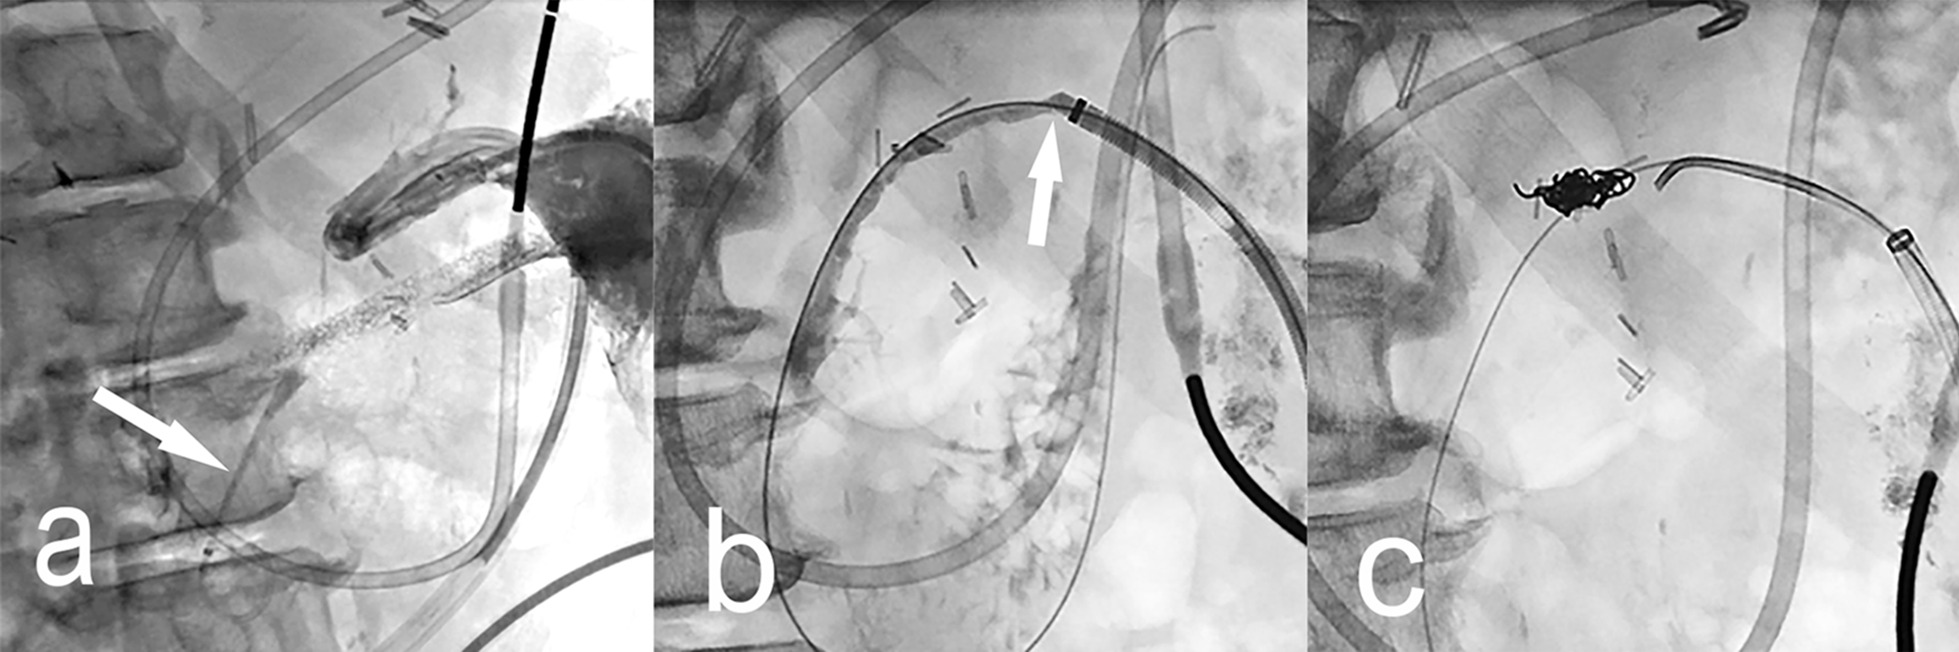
Disconnected pancreatic duct syndrome managed with transcavitary duct stent placement. Patient 8 presented with severe necrotizing pancreatitis. a Coronal reconstruction of abdominal CT scan shows a very large peri-pancreatic walled-off necrosis (WON). b Abdominal radiograph during contrast injection into the WON cavity 2 weeks of drainage shows improvement but persistence of amorphous filling defects caused by debris (arrowheads). c Radiograph during percutaneous necrosectomy shows a forceps introduced through a peel-away sheath grasping the large conglomerates of necrotic and fibrinous material during the process of evacuation. d After resolution of the peri-pancreatic WON a cavitygram shows communication with the pancreatic duct (arrowheads) through a focal disruption (curved arrow) at the neck of the pancreas. e Plastic stent in transit through the percutaneous tract is faintly visible as it is advanced in a folded configuration (arrowheads) over parallel guidewires, one directed towards the pancreatic tail and the other through the ampulla. f CT scan shows the plastic stent appropriately positioned in the pancreatic duct bridging the head and tail segments of pancreatic duct. After one month the stent was removed endoscopically and 6 months later the patient had no recurrence of peri-pancreatic abnormalities and no need for pancreatic enzyme supplementation
